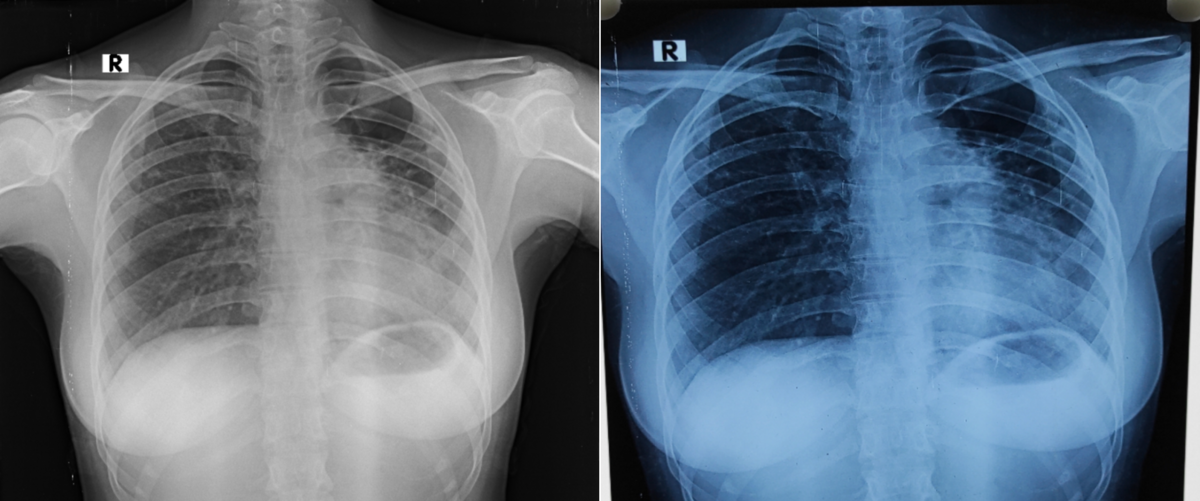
A digital CXR image (on the left) viewed in a DICOM viewer and its corresponding smartphone-captured photo (on the right). CXR: chest x-ray; DICOM: Digital Imaging and Communications in Medicine.
